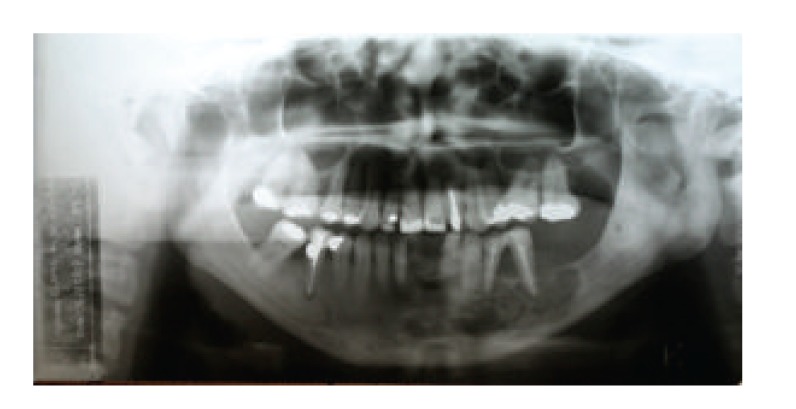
X-ray showing the lesion limits.
